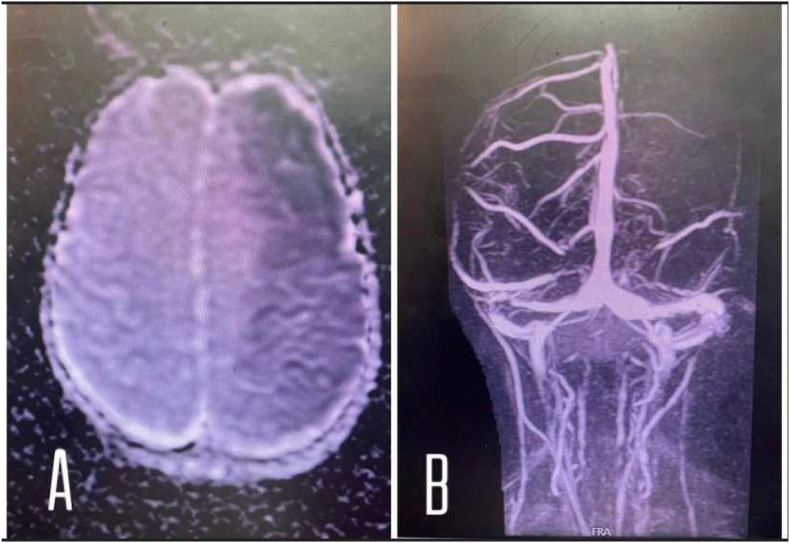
A: MRI shows extensive infarction of subacute aspect (ill-defined vague hypodensity), in the territory of the left middle cerebral artery without hemorrhagic transformation and no significant mass effect. B: MRA shows the occlusion of the left middle cerebral artery.
